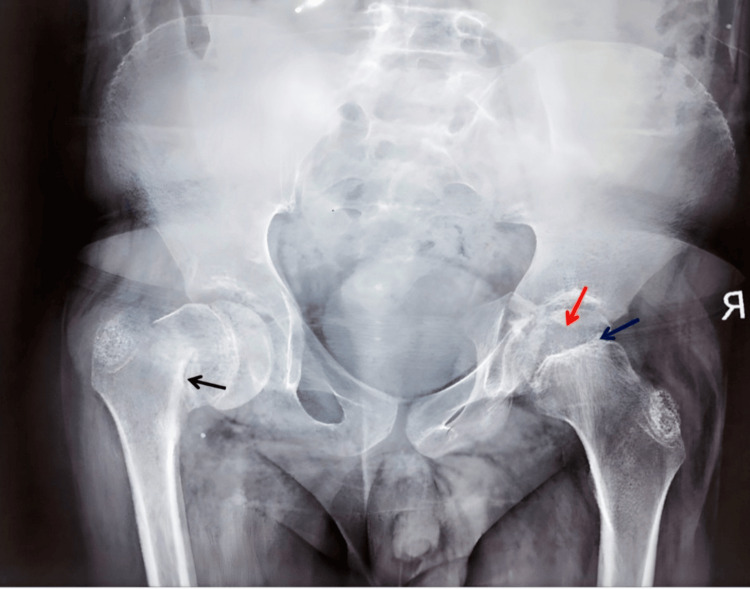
Pelvic radiograph showing a small, sclerosed femoral head (red arrow) with an irregular, sclerotic epiphysis (blue arrow), consistent with avascular necrosis, and a pathological fracture of the left femoral neck (black arrow)
